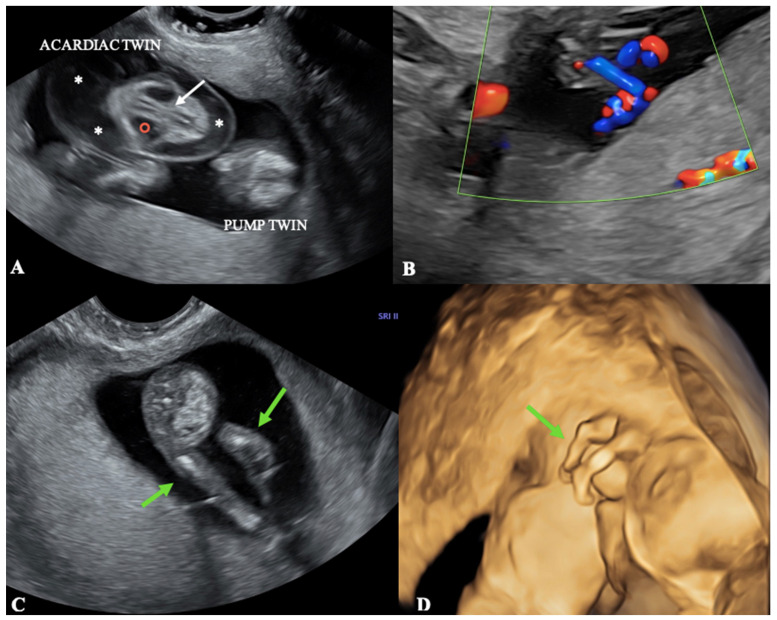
A transabdominal ultrasound in a 14-week pregnant patient. (A) In the 2D grayscale image, the monster twin appeared bigger when compared to the previous ultrasound, so the rudimental vertebral column (white arrow), the anechoic cyst (red circle), and the edema of soft tissues (*) were more evident. (B) The color Doppler study showed a marked perfusion into the umbilical cord of the acardiac fetus. (C) A 2D image showing the lower limbs of the acardiac twin (green arrows). (D) A 3D picture showing the lower limbs of the acardiac (green arrow) over the twin pump’s back.
